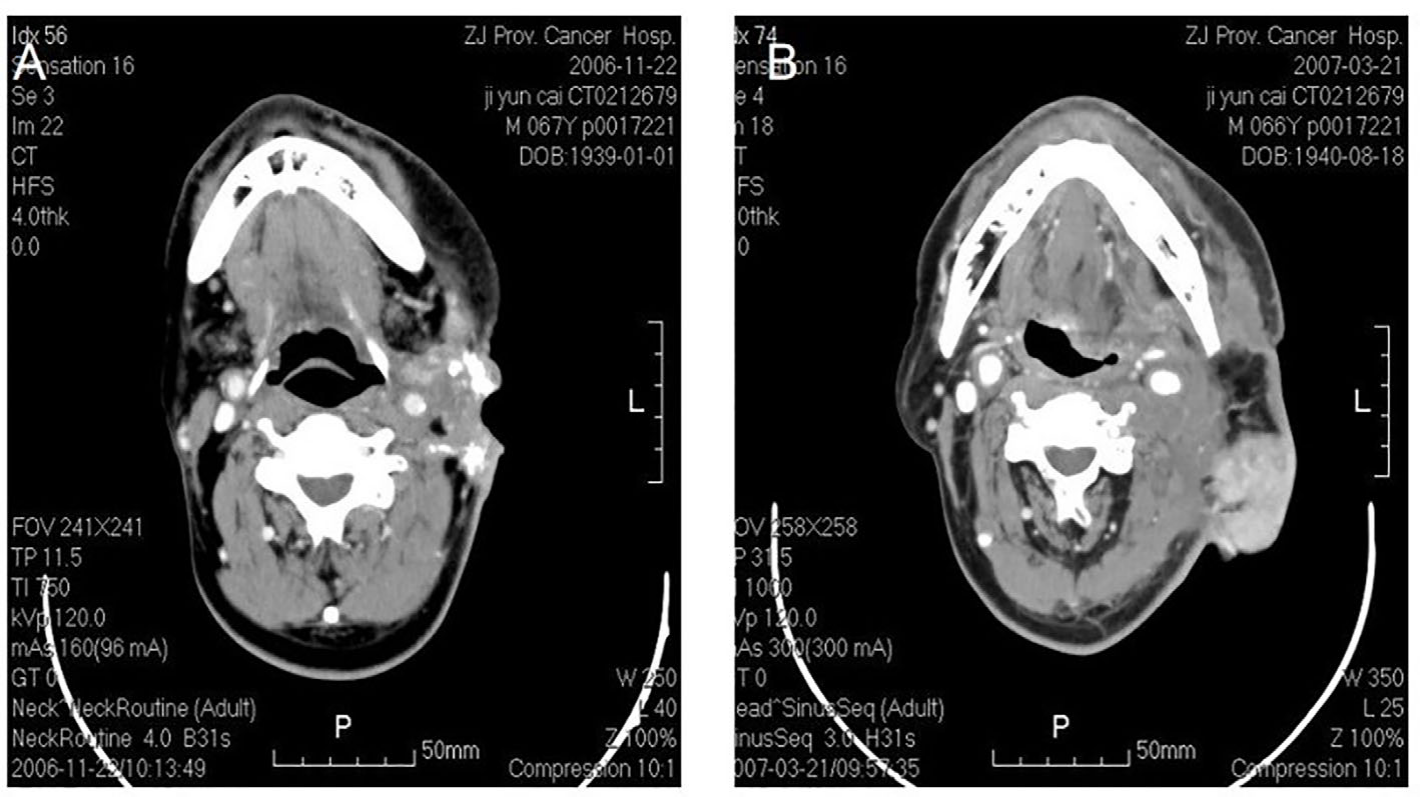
Osteosarcoma in left neck. CT shows an irregular soft tissue imaging in the left neck, with heterogeneited density, several schistose calcification in it. CT also shows that the lesion has been heterogeneited enhanced after contrast, with obscure margin to the left cervical vascular, with superficial elcosis. (A) The lesion recurred after 4 months. (B) The picture on the right shows pedicled pectoralis major myocutaneous flap with surrounded recurrent lesions.
